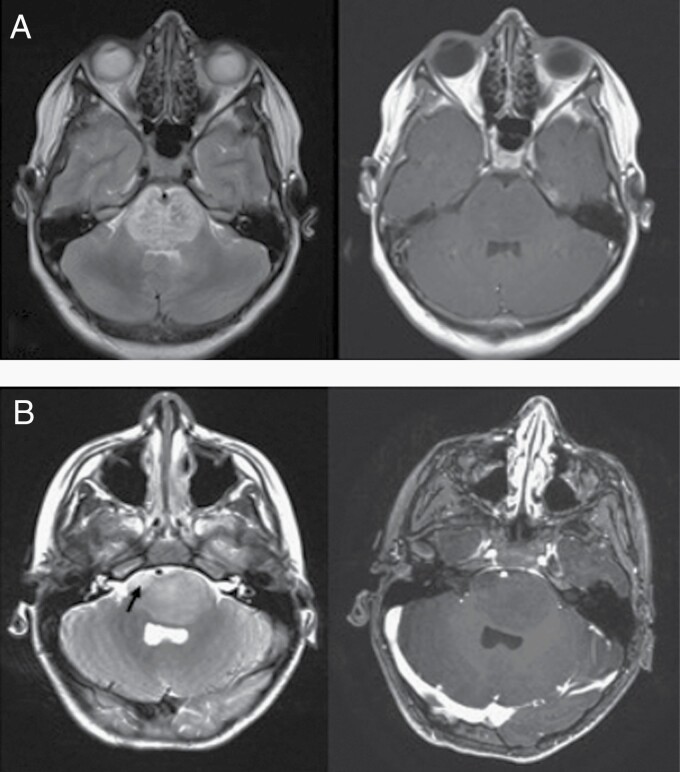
A visual example of MRI radiomics. Example axial T2 (left) and T1 (right) MRI for 2 children. T2-weighted MR images of a patient who survived 20 months (A) and a patient who survived only 3 months (B) show heterogeneous or coarse intensity distribution with punctate foci of dark signal interspersed within T2 hyperintensities in the patient (A) compared to (B), where more confluent intensities are seen with a more localized T2 hyperintense soft tissue abnormality in right anterior pons (arrow). The T1 post-contrast MRI demonstrates limited qualitative characteristics of the tumor.
